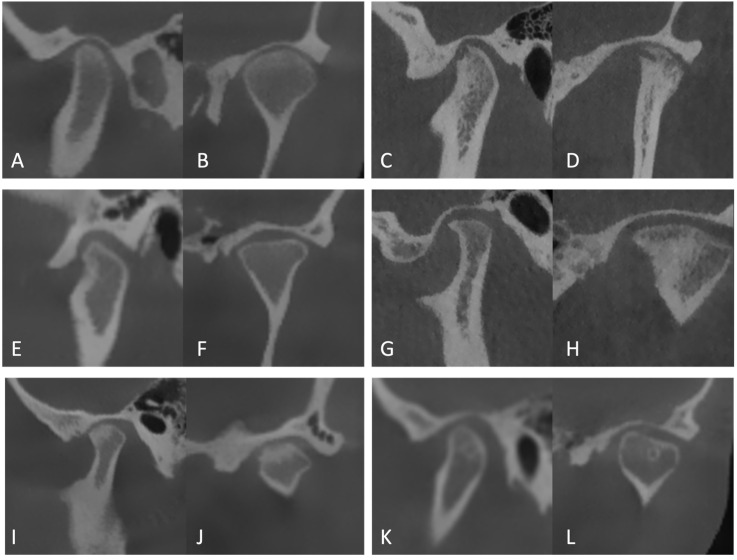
CBCT images of the mandibular condyles demonstrating normal morphology (A,B), erosion (C,D), flattening (E,F), osteophyte (G,H), sclerosis (I,J), and subchondral bone cyst (K,L) in sagittal and coronal views.
